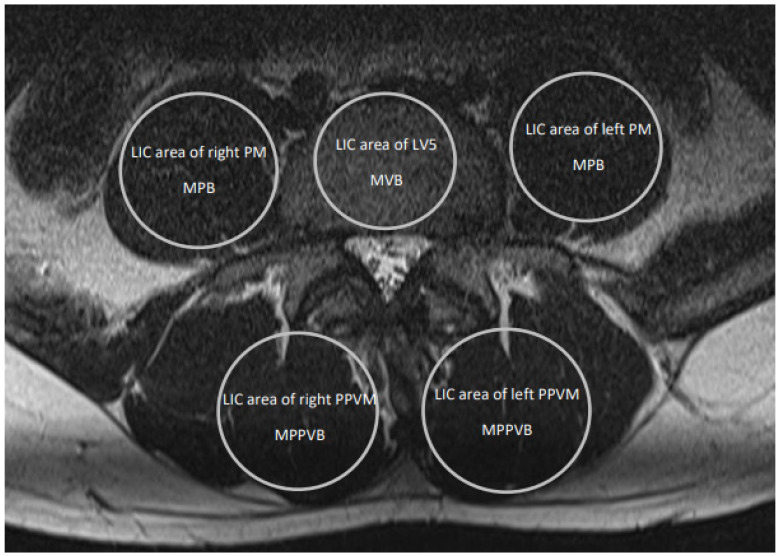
Example showing measurement schemes of LIC area size and brightness in the fifth lumbar vertebral body, with both psoas and both erector spinae muscles. Axial MRI slice through the lower lumbar spine showing vertebral and muscle structures of a 39-year-old man without spinal pathologies. LIC indicates largest inscribed circle; MRI, magnetic resonance imaging; L5, lumbar vertebra V; PM, psoas muscle; PPVM, posterior paravertebral muscle; MVB, mean vertebral (body) brightness; MPB, mean psoas (muscle) brightness; MPPVB, mean posterior paravertebral (muscle) brightness.
